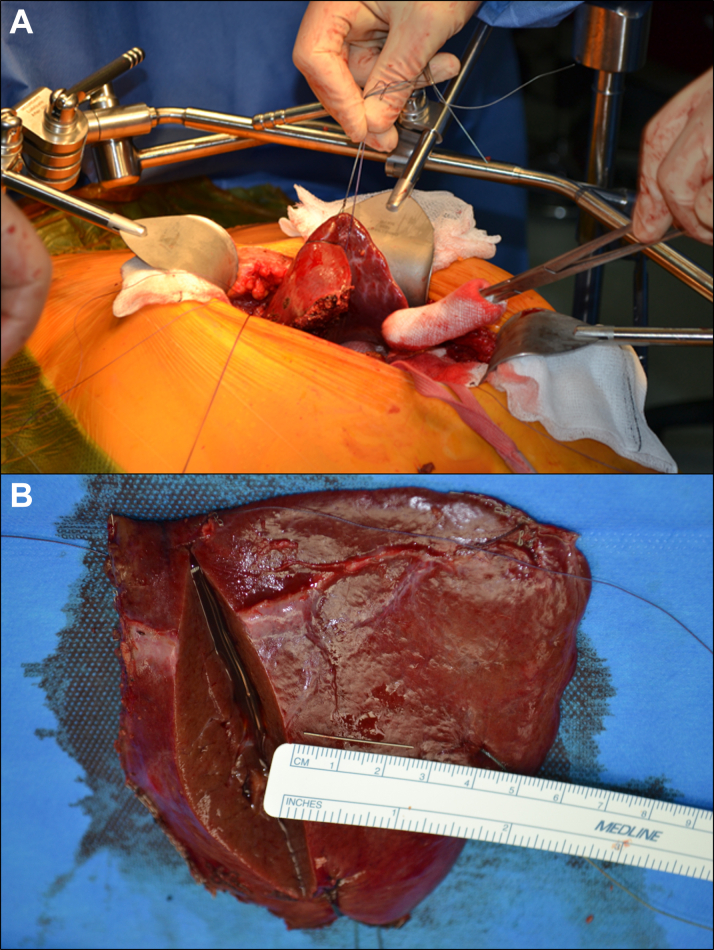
Partial Hepatectomy Partial hepatectomy was performed given that the needle was not easily extractable despite localization with ultrasound during surgery (A). The needle is subsequently shown once removed from the resected segment (B).
